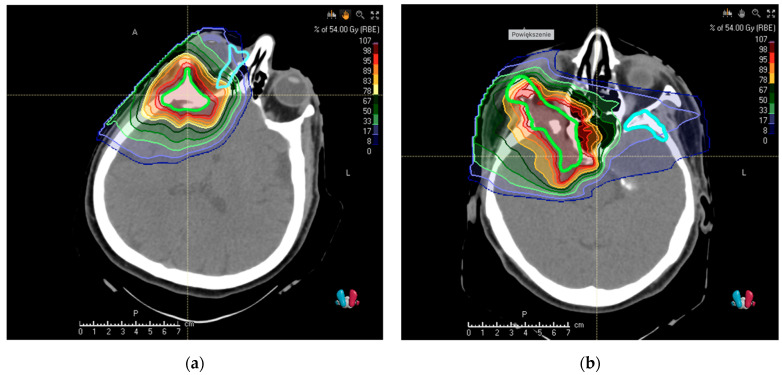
Display of dosimetric consequences of omission of tumor volume in two cases in which PET/CT delivered new crucial information in addition to CT + MRI. Thick fluorescent contours represent volumes of observers performed using MRI only, thick light blue contours display the newly identified tumor extent: (a) intraorbital (b) in the left sphenoid bone. The treatment plans were carried out for MRI volumes only.
